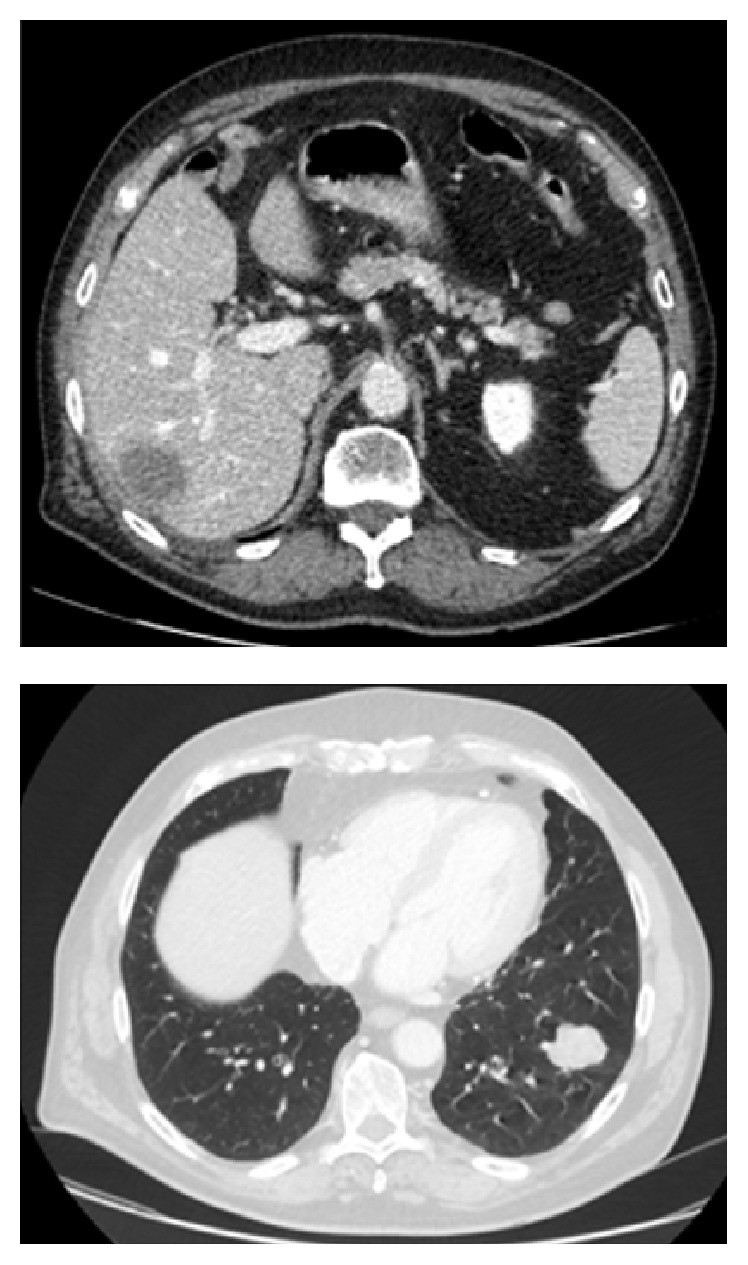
Computed tomography scan of the patient of case 1 at the diagnosis of metachronous hepatic and pulmonary metastases.
